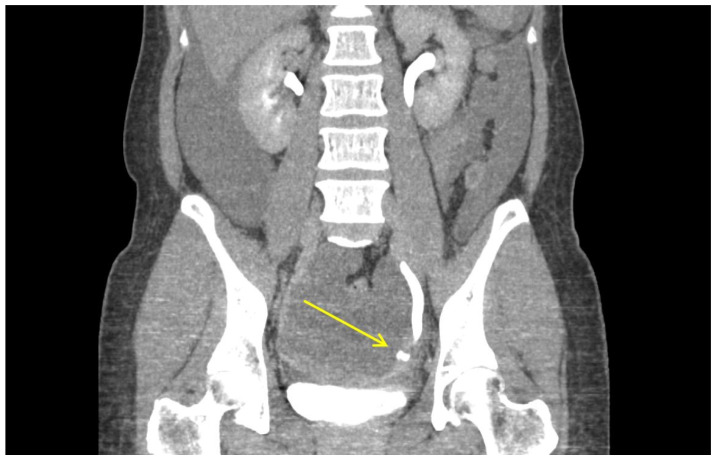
Sagittal image of urography CT (computed tomography) showing left distal ureteral contrast leakage with profuse amount of fluid collection in the abdominal cavity.
